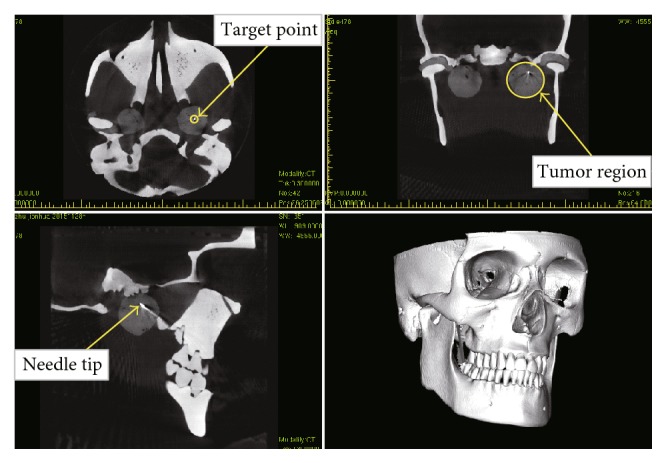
Postoperative CT scan results.
